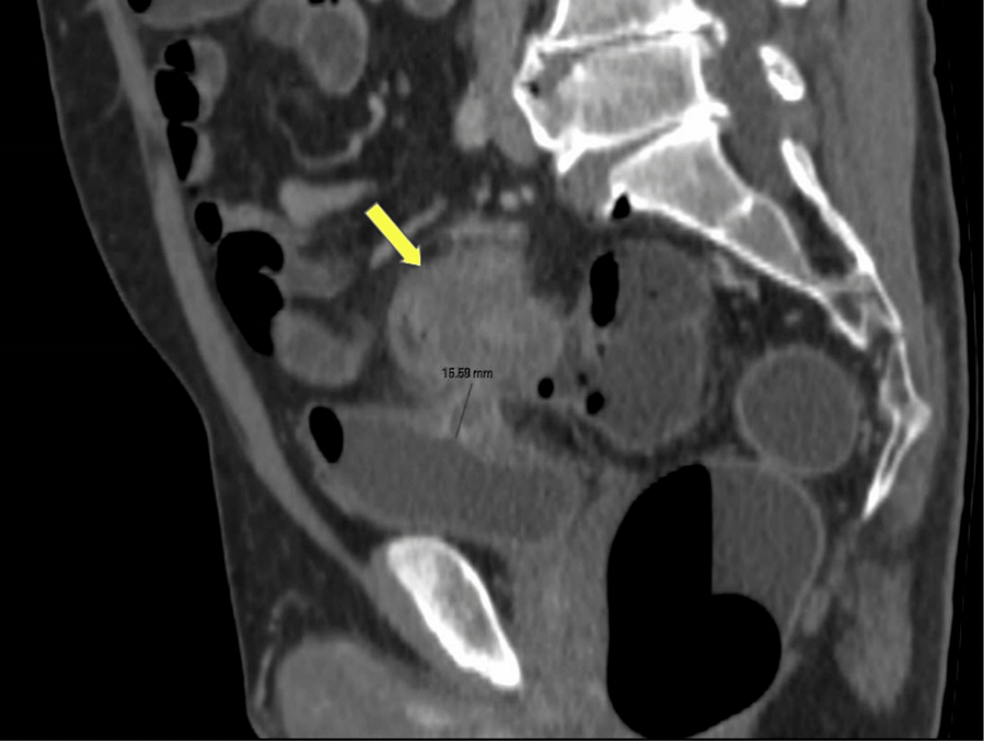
Sagittal view of the abdominal CT scan showing the mass in the sigmoid colon (arrow) and the colovesical fistula (annotation). CT: Computed tomography.
